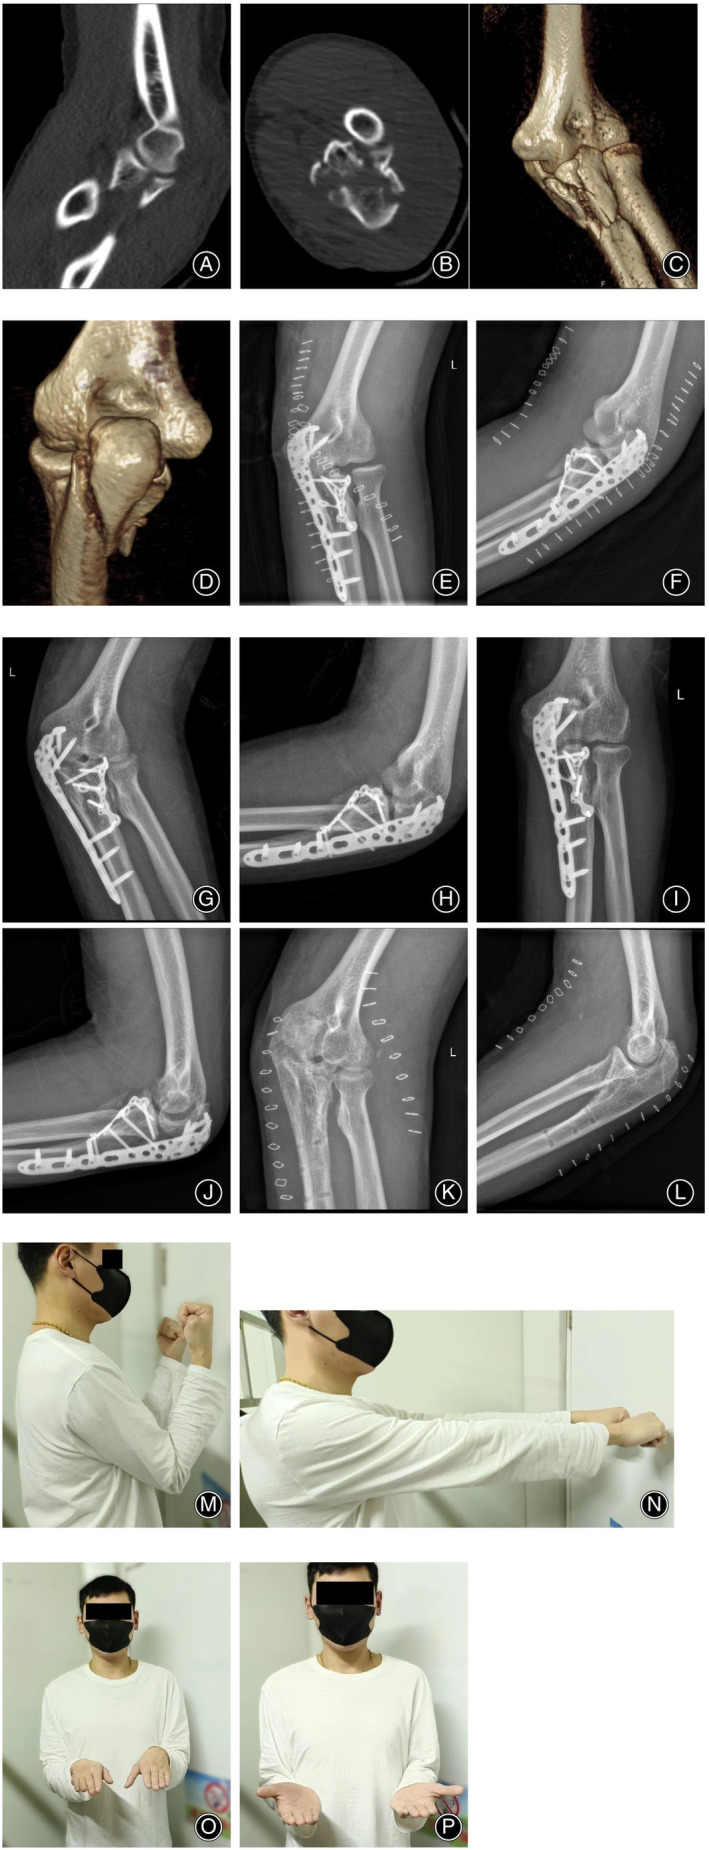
Typical case 2. A 38‐year‐old man suffered from fall‐related injuries. (A–D) Preoperative CT images showed ulnar coronoid process fracture and olecranon fracture. (E, F) Postoperative x‐ray images showed an anatomical reduction of the coronoid process fracture and olecranon fracture. (G, H) Ten weeks after the operation, the radiographs of the elbow joint showed that the fracture was not displaced. (I, J) Seventeen months after the operation, internal fixation was in place and the bone has healed. (K, L) Twenty‐two months after the operation, the internal fixation was removed. (M–P) The function of the patient's elbow joint at the last follow‐up was favorable, and the patient was pain free.
